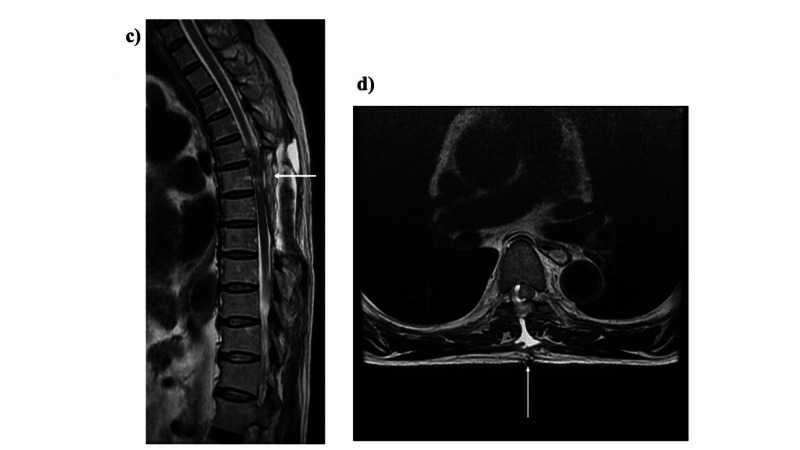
Thoracic spine intradural extramedullary tumor (Th6). Postoperative images. c) Sagittal plane of thoracic spine MRI: This image shows the removal of an extramedullary tumor, with the spinous processes in the Th5 to Th8 region removed. The arrows indicate where the tumor was removed. d) Transverse of thoracic spine MRI: the compressed tumor has been removed, but the spinal fluid was leaking.
